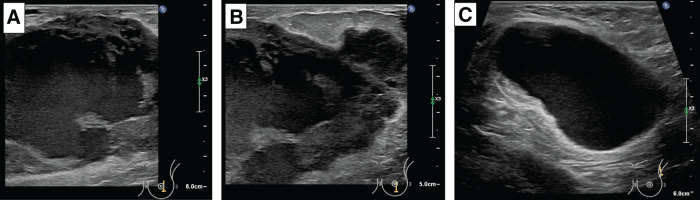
Initial US. Internal heterogeneity of the tumor and the presence of halo findings are noted. (A) Central portion of the left breast tumor, (B) caudal marginal portion of the left breast tumor, (C) swelling of axillary lymph nodes. These findings are the first indication of the presence of a malignant tumor with a mixed internal component and lymph node metastasis. US, ultrasonography
